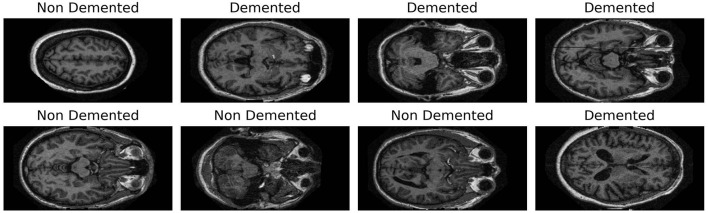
Representative structural MRI slices comparing healthy and Alzheimer's disease cohorts.
